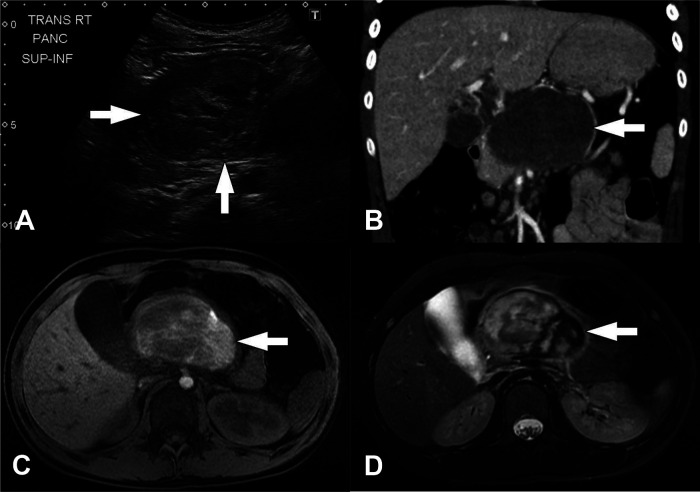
Solid pseudopapillary neoplasm. (A) Transverse image from initial transabdominal ultrasound shows a heterogeneous mass (arrows) in the expected location of the pancreas. (B) Coronal reformatted image from subsequently performed CT of the abdomen obtained in the portal venous phase following intravenous contrast material administration shows a heterogeneously hypoattenuating mass arising from the body of the pancreas (arrow). Subtle enhancing septations/material are visible within the mass. (C) Axial T1-weighted and (D) axial T2-weighted images from subsequently performed MRI show the mass in the body of the pancreas (arrows). The mass is internally heterogeneously T1-weighted and T2-weighted hyperintense with prominent peripheral T2-weighted hypointensity. These signal characteristics suggest the presence of internal hemorrhage.
